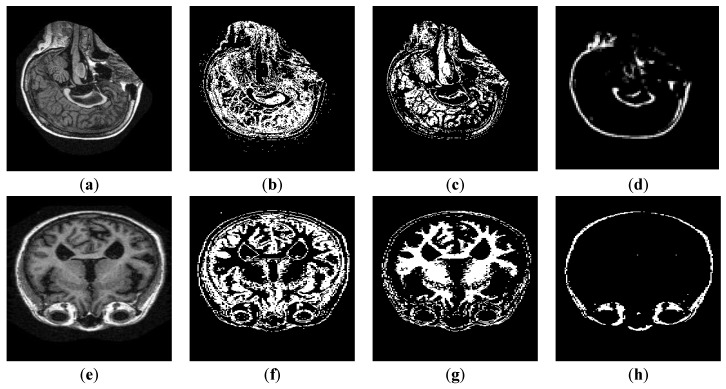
(a,e) Input-enhanced MRI. (b,f) GM segment of input MRI. (c,g) WM segment of input MRI. (d,h) CSF segment of input MRI.
